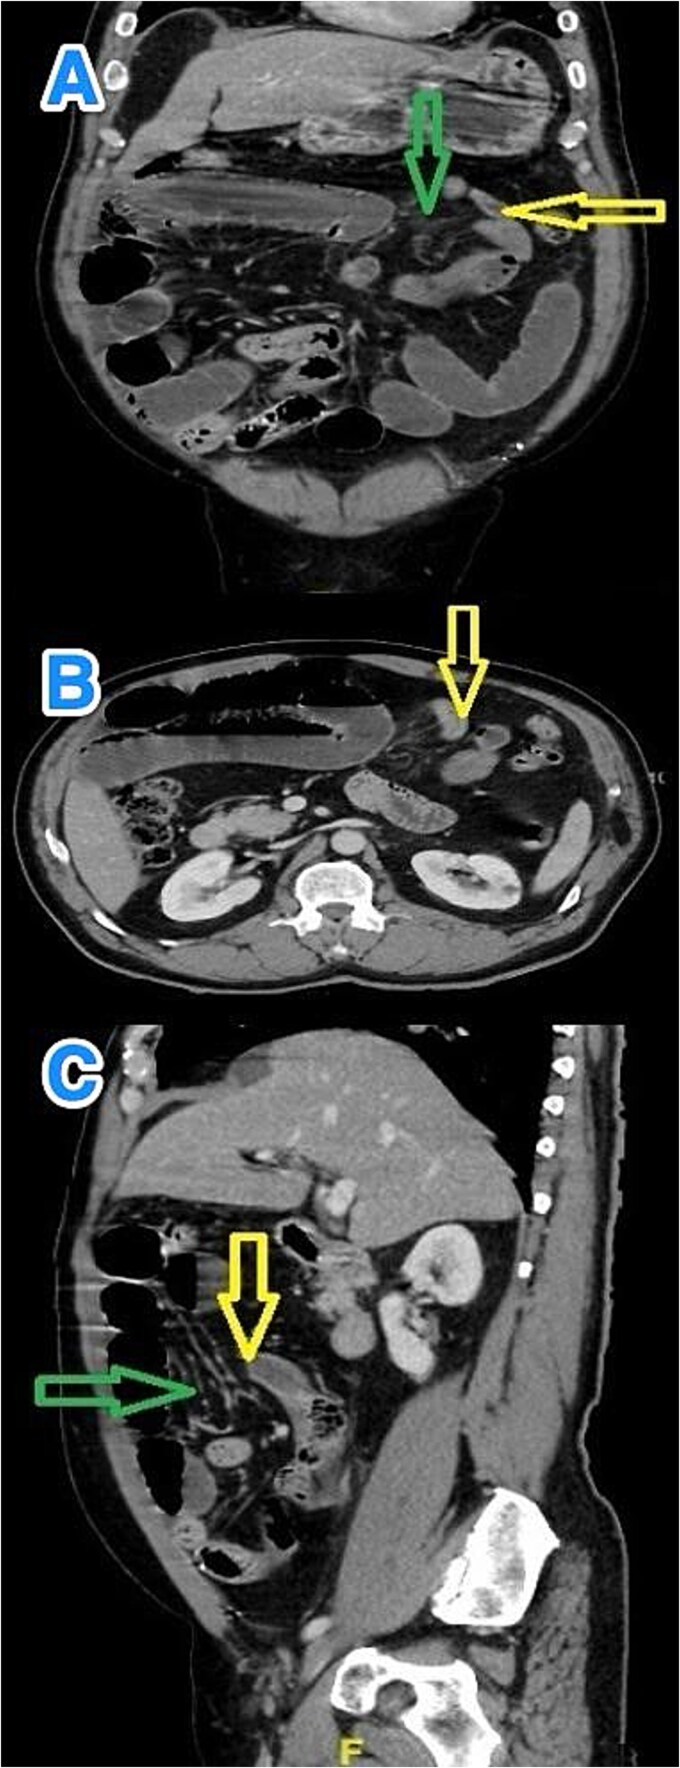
Multiview contrast-enhanced CT images of the abdomen demonstrate: (A) a coronal view showing herniated bowel (yellow arrow) through the greater omentum (green arrow), (B) an axial view showing the transition zone (yellow arrow), and (C) a sagittal view showing herniated bowel (yellow arrow) through the greater omentum (green arrow).
